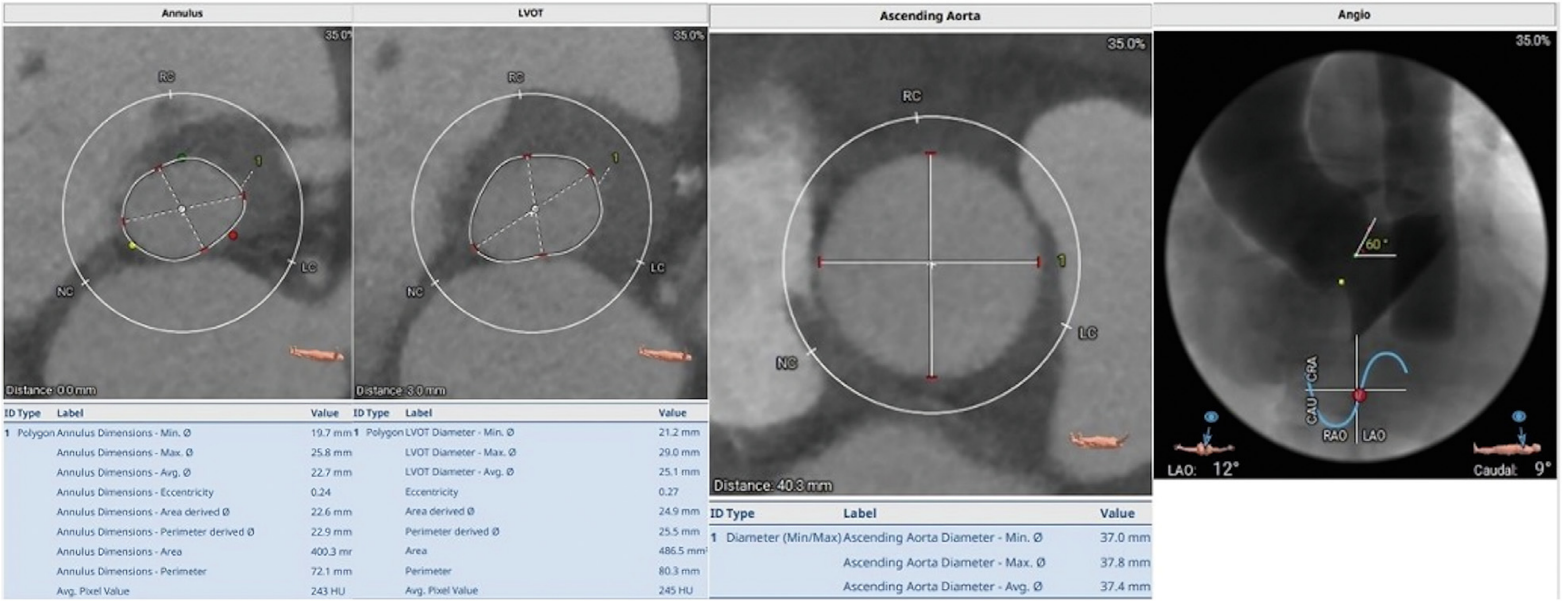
Patient 2: Preprocedural computed tomography. LVOT, left ventricular outflow tract.
